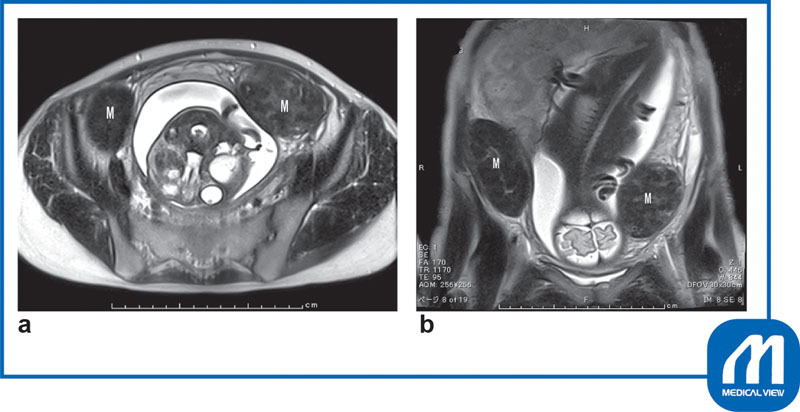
Case 1: magnetic resonance imaging findings (T2W). Two myomas (M) (right 9 × 7 × 5 cm, left 11 × 7 × 6 cm) were found slightly above the uterine isthmus.
